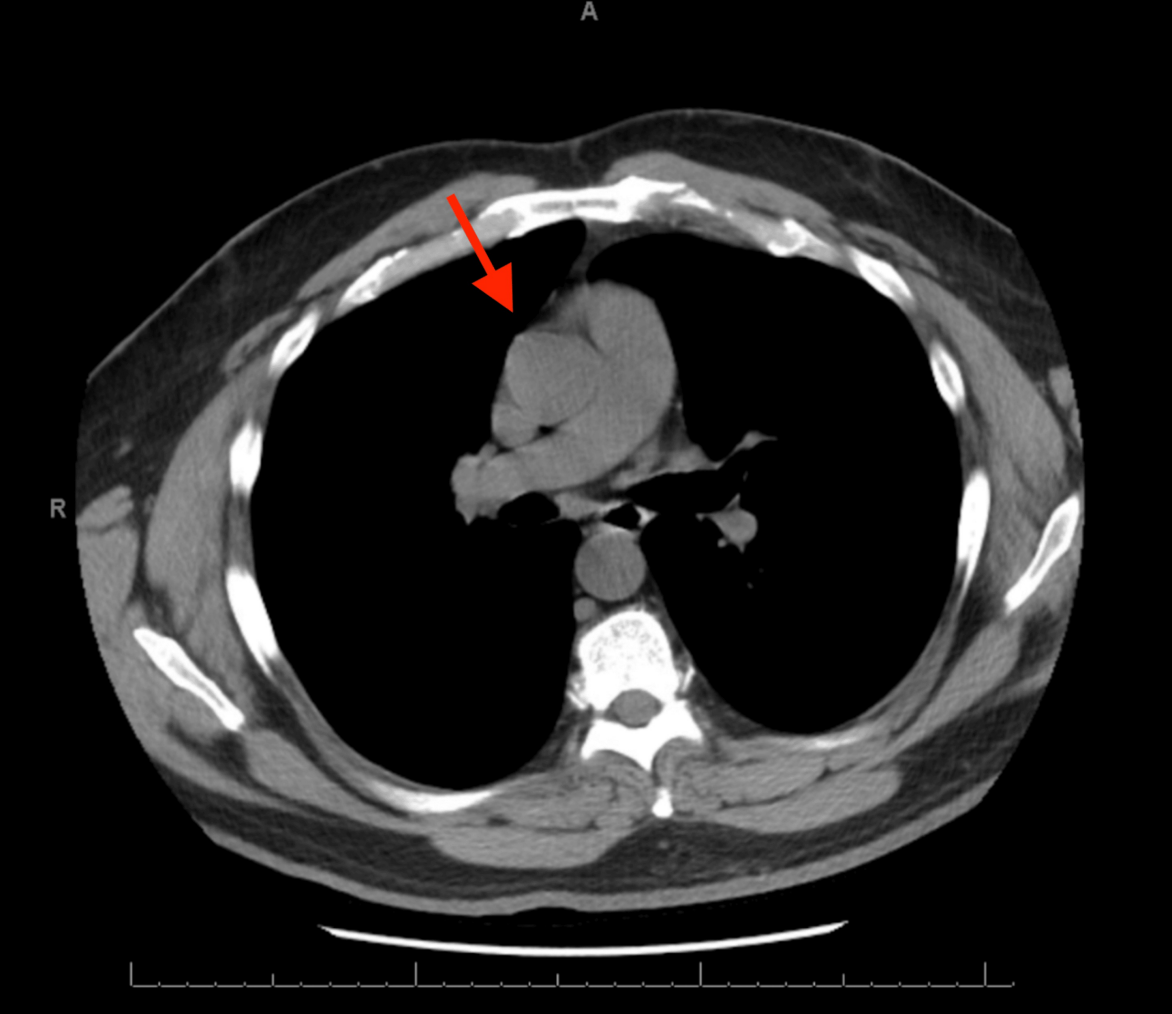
Axial CT image from 2019 demonstrating an incidental right-sided aortic arch (red arrow) without significant tracheal compression. CT: computed tomography
